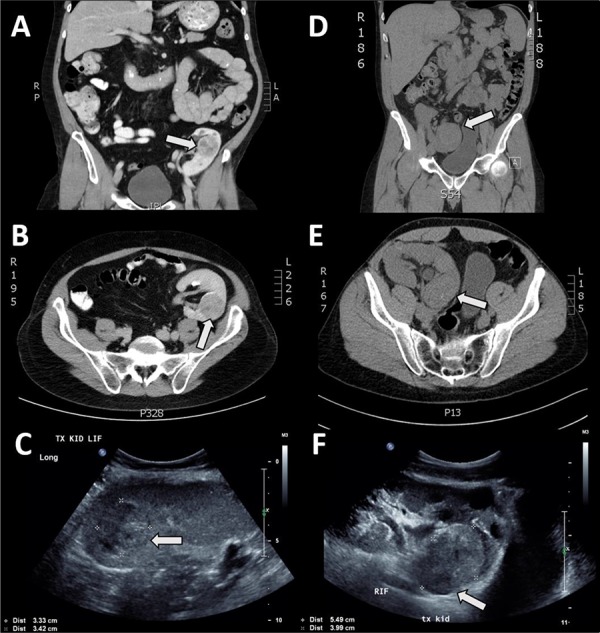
A. Computer tomographic scan (CT) case 1: coronal section showing transplant kidney in left iliac fossa; distinct mass present in upper pole and enhanced with contrast, designated by arrow. B. CT case 1: transverse section showing distinct mass in transplant kidney enhanced with contrast, predominantly posteriorly located. C. Ultrasound case 1: showing distinct mass within kidney. D. CT case 2, coronal section showing transplant kidney in right iliac fossa; indiscriminate mass located in lower pole without contrast enhancement. E. CT case 2, transverse section showing indiscriminate mass in posterior portion of kidney. F. Ultrasound case 2, showing distinct mass in posterior portion of kidney cortex.
